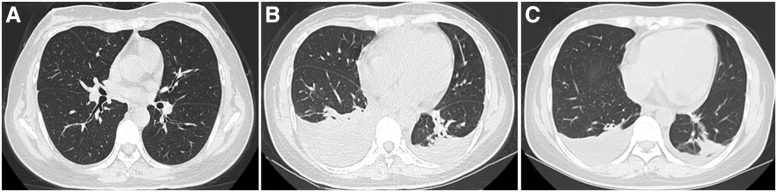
CT images of the lung. (A) lung CT scan on October 26, 2024 showing small nodules in both lungs without pleural effusion; (B) lung CT scan on November 5, 2024 showing pneumonia, bilateral pleural effusion, lymphadenopathy, as well as mild pericardial effusion; (C) lung CT scan on November 12, 2024 indicating the improvement of pneumonia and pleural effusion. CT = computed tomography.
